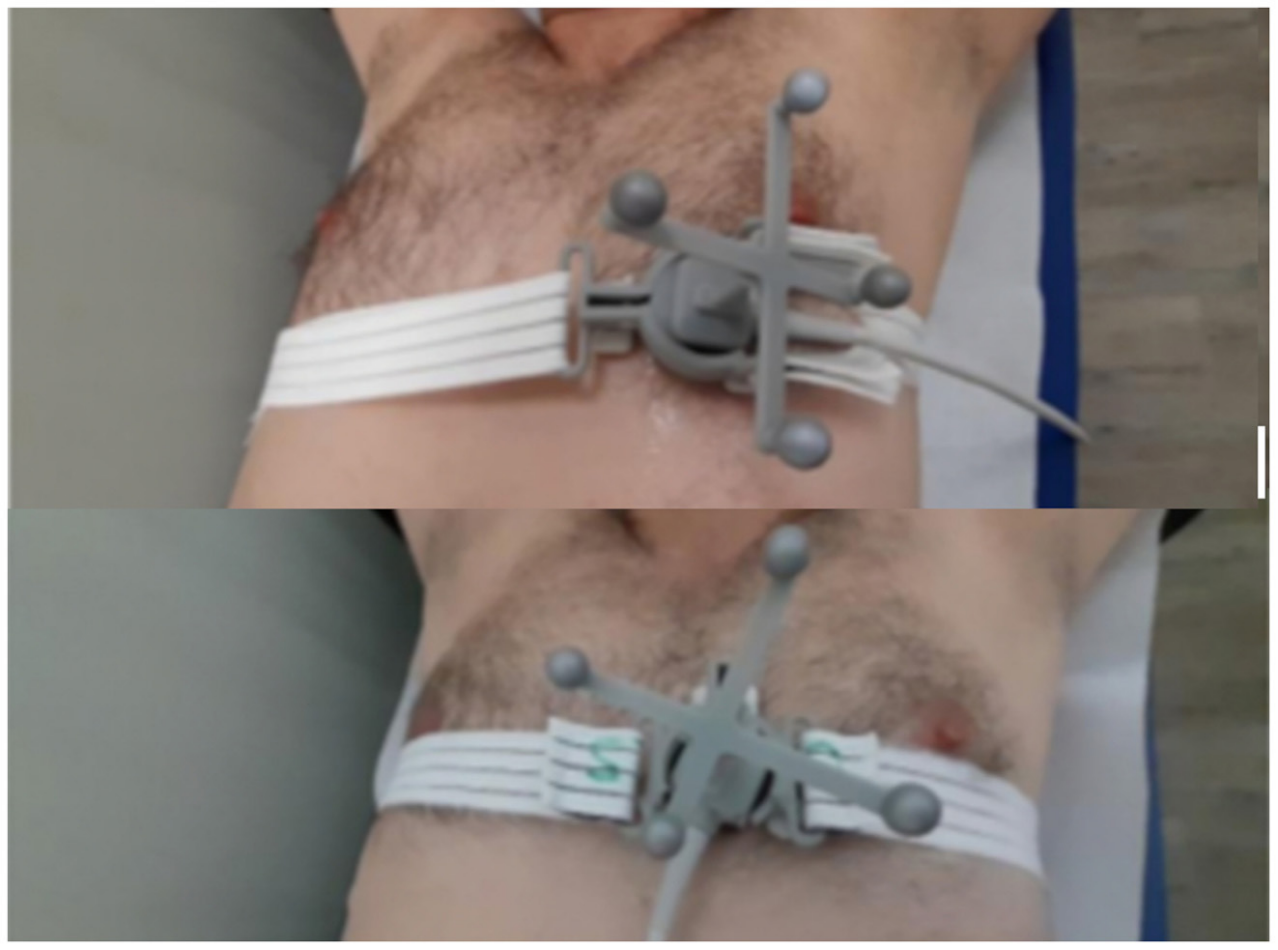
Images of the ultrasound probe housed in the holder containing the markers for optical localization. The probe and the support are kept adherent to the patient's chest by means of an adjustable elastic band. Upper panel apical position; Lower panel parasternal position.
